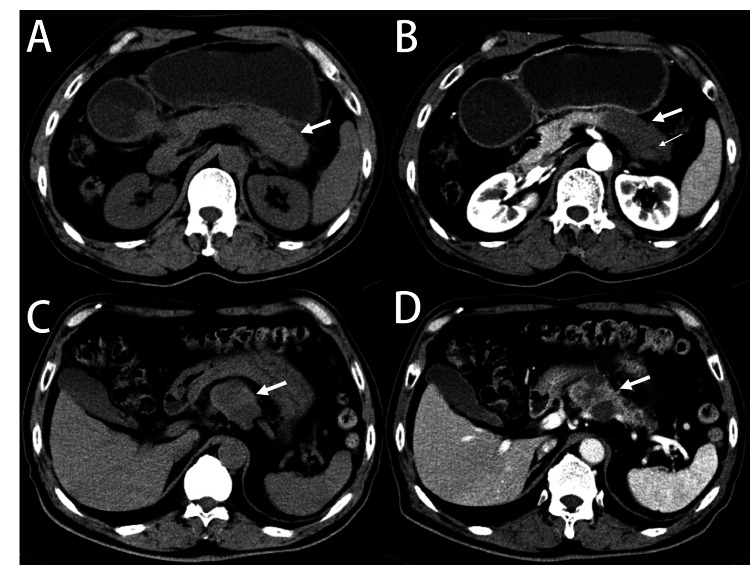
Undifferentiated carcinoma with osteoclast-like giant cells appeared as cystic and solid lesions. (A) A 60-year-old man with undifferentiated carcinoma with osteoclast-like giant cells in the tail of the pancreas. The volume of a pancreatic tail was increased (white arrow). (B) An enhanced CT scan showed a major solid lesion with hypo enhancement (thick arrows) and a small cystic lesion (thin arrows) was visible. (C) Another 76-year-old man with undifferentiated carcinoma with osteoclast-like giant cells in the tail of the pancreas. Mixed-density lesions (white arrows) are seen in the tail of the pancreas. (D) An enhanced CT scan showed that the major cystic lesions were not enhanced, and the solid nodule at the edge of the lesion was enhanced. CT: computed tomography
